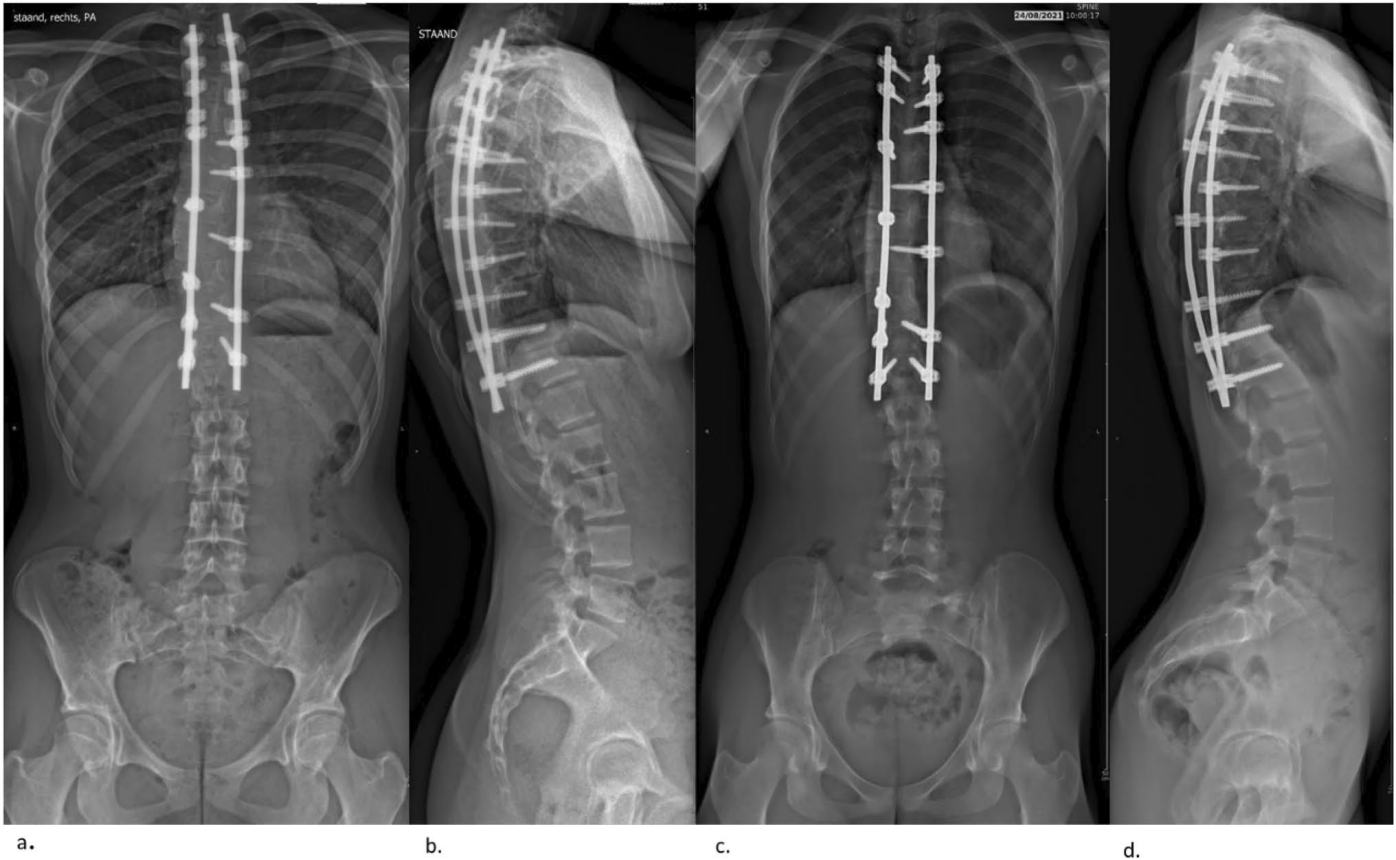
Two-year outcome radiographs. a. AP view claw-construct b. lateral view claw-construct c. AP view all-screw construct d. lateral view all-screw construct
